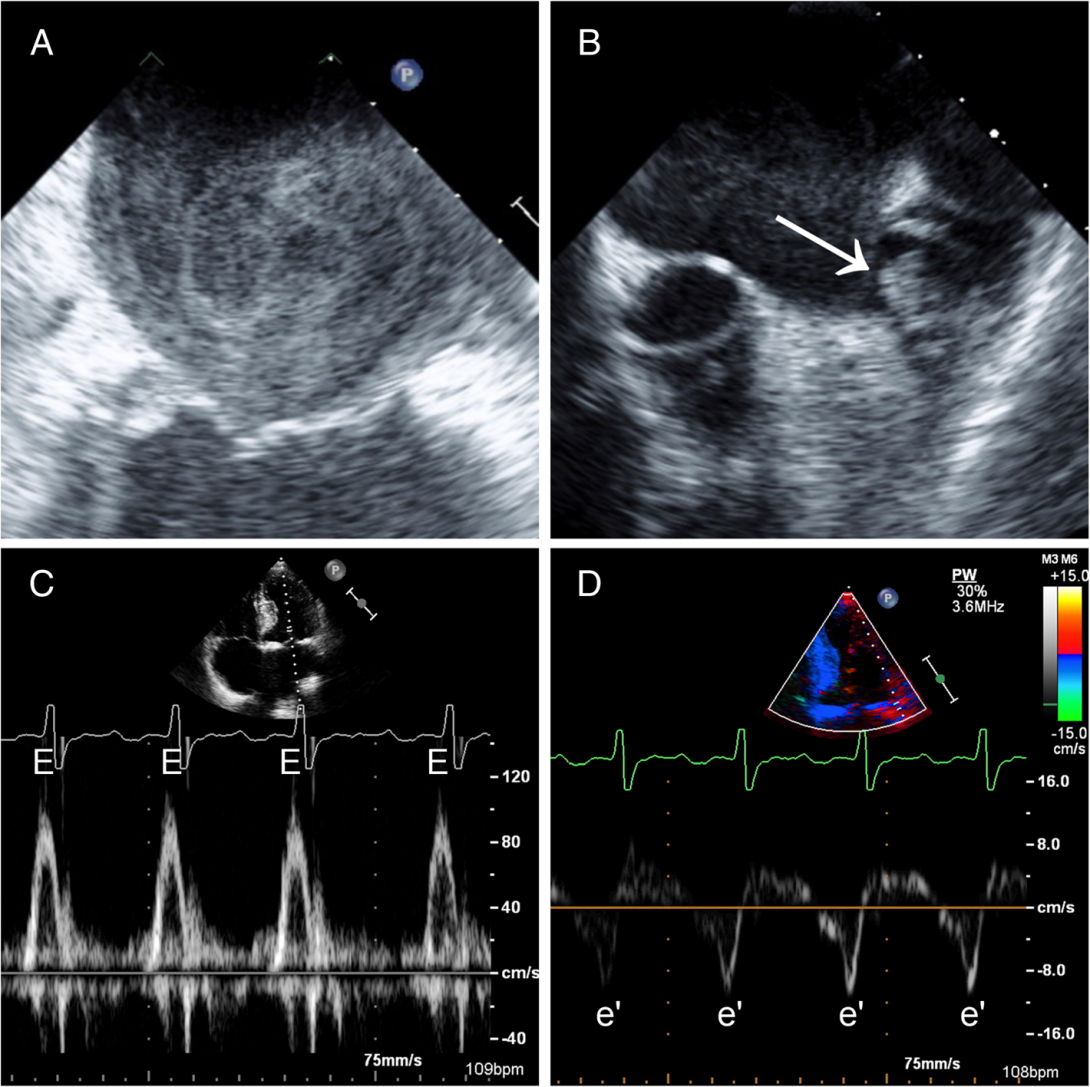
Representative examples. A: left atrial spontaneous echo contrast (SEC). B: left atrial appendage thrombus (arrow). C: Pulsed-wave Doppler recording of early diastolic mitral inflow velocity (E). D: Spectral tissue Doppler imaging of the lateral mitral annulus early diastolic velocity (e’).
